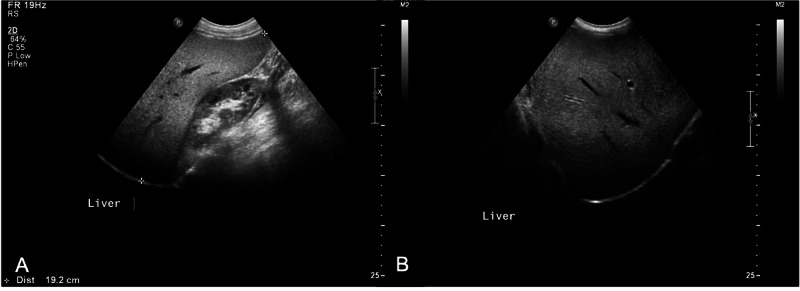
Abdominal ultrasound of patient in case 2 (A) Longitudinal gray scale ultrasound view shows hyperechoic liver (liver steatosis) and normal right kidney. The liver span is enlarged, measuring 19.2 cm in the midclavicular line. (B) Transverse view shows hyperechoic liver.
